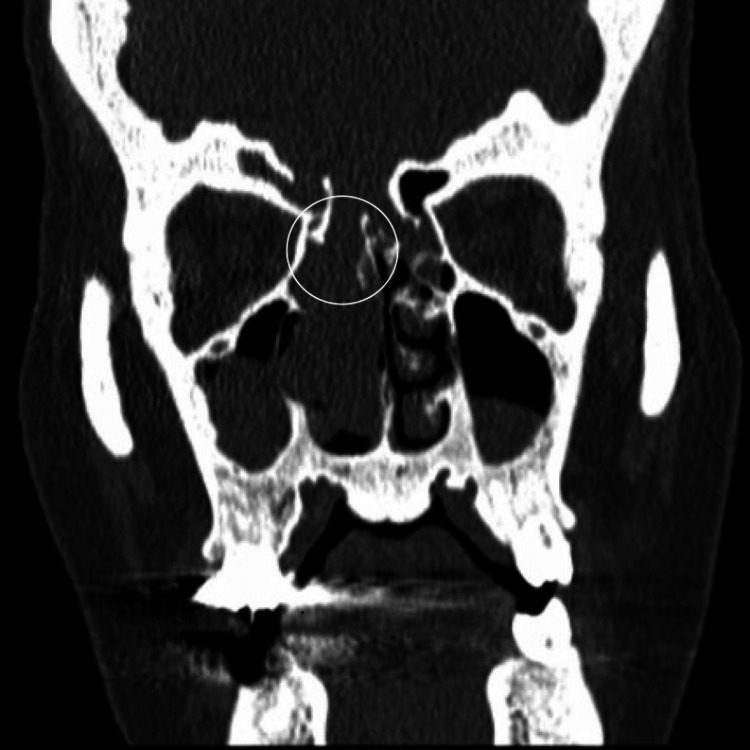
CT scan (coronal view) showing a skull base defect at the right cribriform plate (white circle) with encephalocele filling the right nasal cavity
